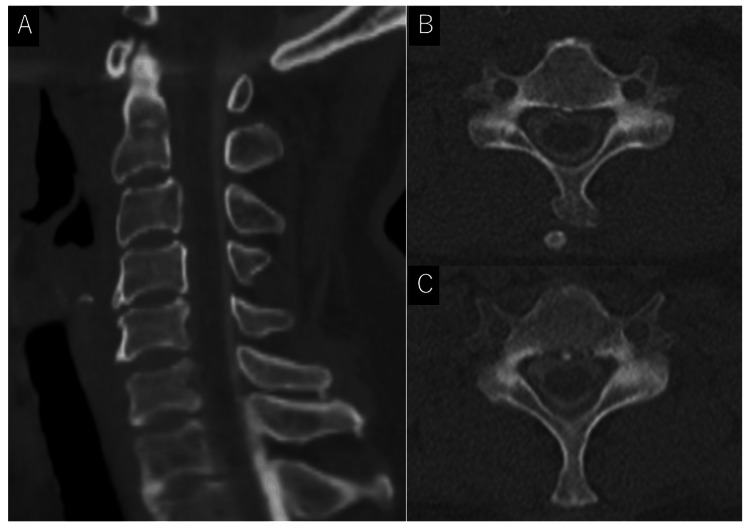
Cervical computed tomography myelography. (A) Sagittal view and (B: C5 level and C: C6 level) axial views of cervical CT myelography showing no hematoma and no lesions compressing the dural canal within the spinal canal.
